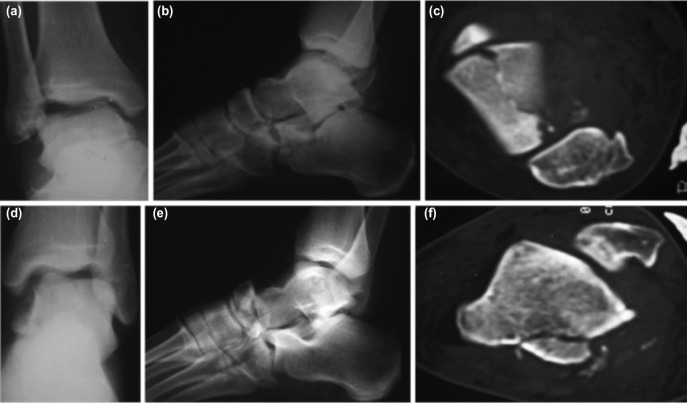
Pre-operative radio-imaging of both feet (a) anterior-posterior radiograph of right ankle showing coronal shear fracture of the body of the talus. (b) lateral radiographs showing anterior process fracture of the calcaneus extending to the calcaneocuboid joint and nondisplaced navicular body fracture. (c) CT scan of the right foot and ankle, showing coronal shear fracture of the body of the talus. (d) anterior-posterior radiograph of left ankle showing subluxation of talus with no obvious fracture. (e) lateral radiographs showing displaced fracture of the navicular body with talo-navicular subluxation. (f) CT scan of the left foot and ankle showing fracture of posteromedial process fracture of the talus.
